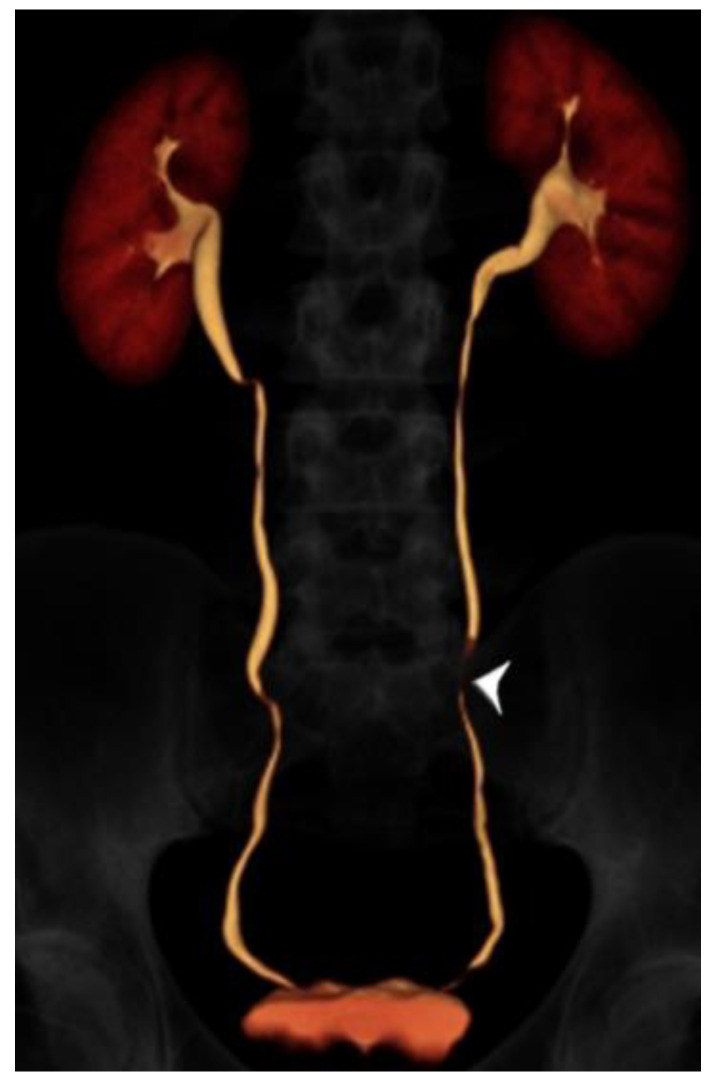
24 year-old male with gross hematuria imaged with split-bolus CT urography. Volume-rendered image of the excretory phase shows excellent opacification of the urinary collecting system. There is a very short region where the mid left ureter is not opacified by contrast (arrowhead). The total table time was 14 min and the dose-length product was 614 mGy·cm.
